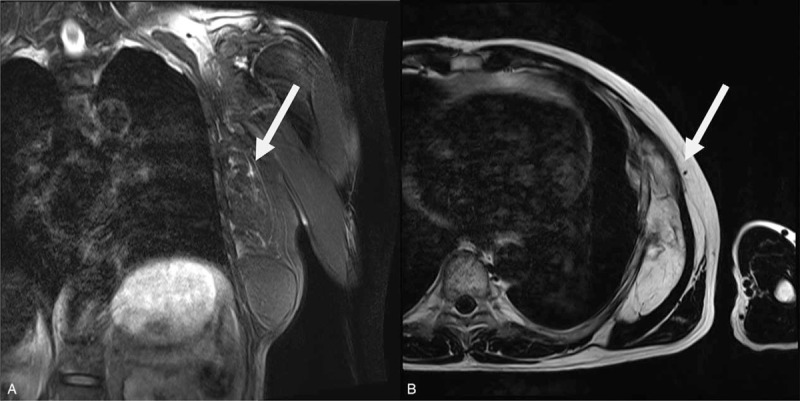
A septate mass measuring approximately 159 x 144 x 38 mm with a high SI on both T1- and T2WIs with fat-suppression in the left chest wall of MRI and protruding inside the thoracic cage, possibly involving the pleura (A: coronal section, B: axial section).
